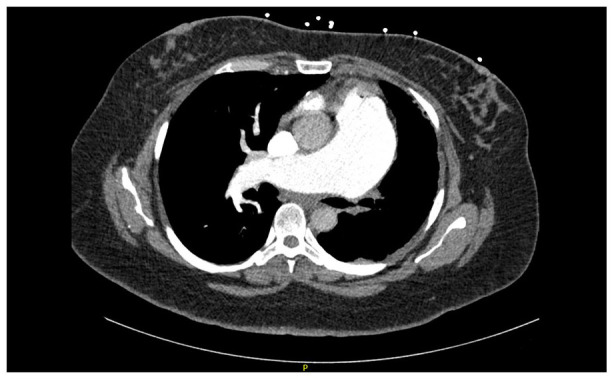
CT angiography chest showing agenesis of left branch of pulmonary artery.
